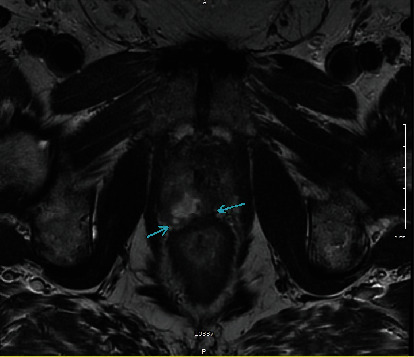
Axial T2-weighted MRI image showing the caudal aspect of the tumor abutting the caudal aspect of the rectum/upper aspect of the anal canal with possible serosal invasion.
